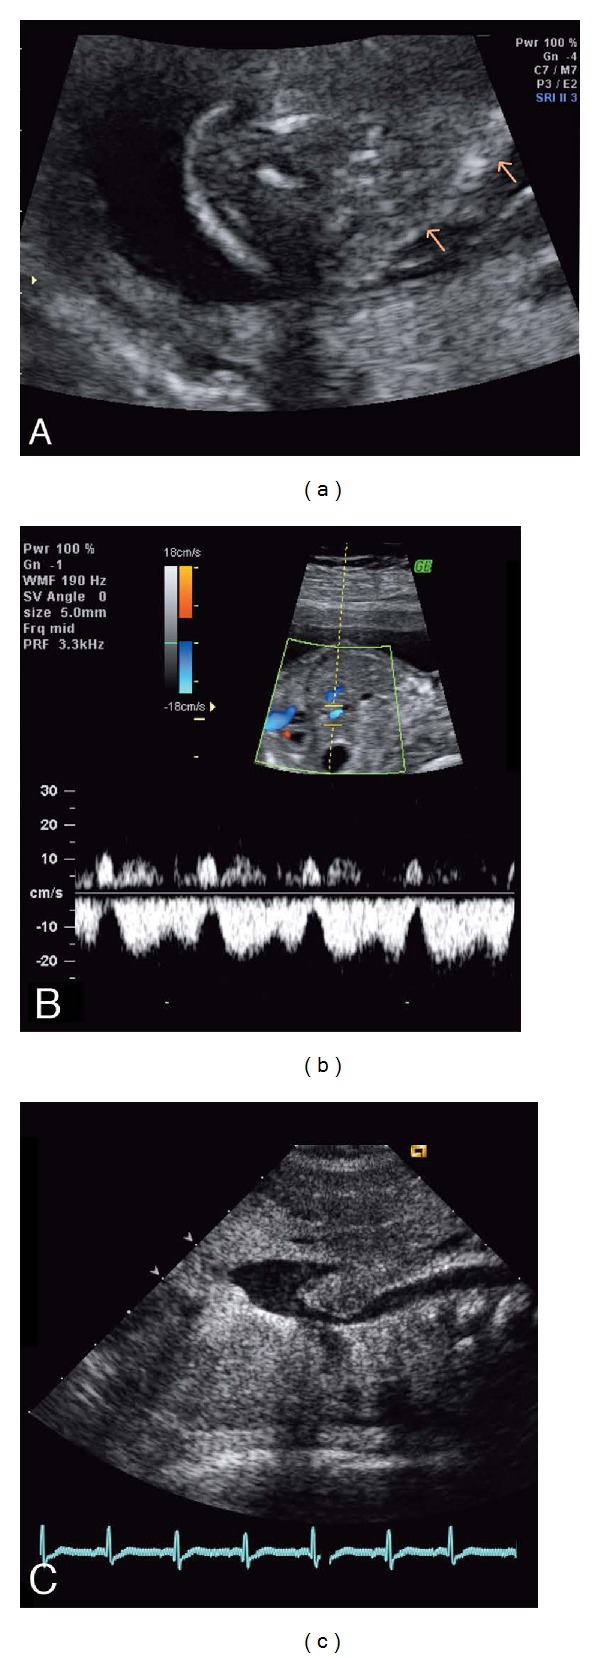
Ultrasound images prenatally and postnatally. (a) Fetal scan at the 12th week of pregnancy demonstrating moderate nuchal edema and cystic hygroma. (b) Pathological flow of the venous duct at the 20th week. During atrial contractions, a reverse flow is noticeable. (c) Two-dimensional echocardiogram of a newborn with absent SVC. The azygos and the hemiazygos veins have fused, and the venous duct formed is draining into the IVC.
